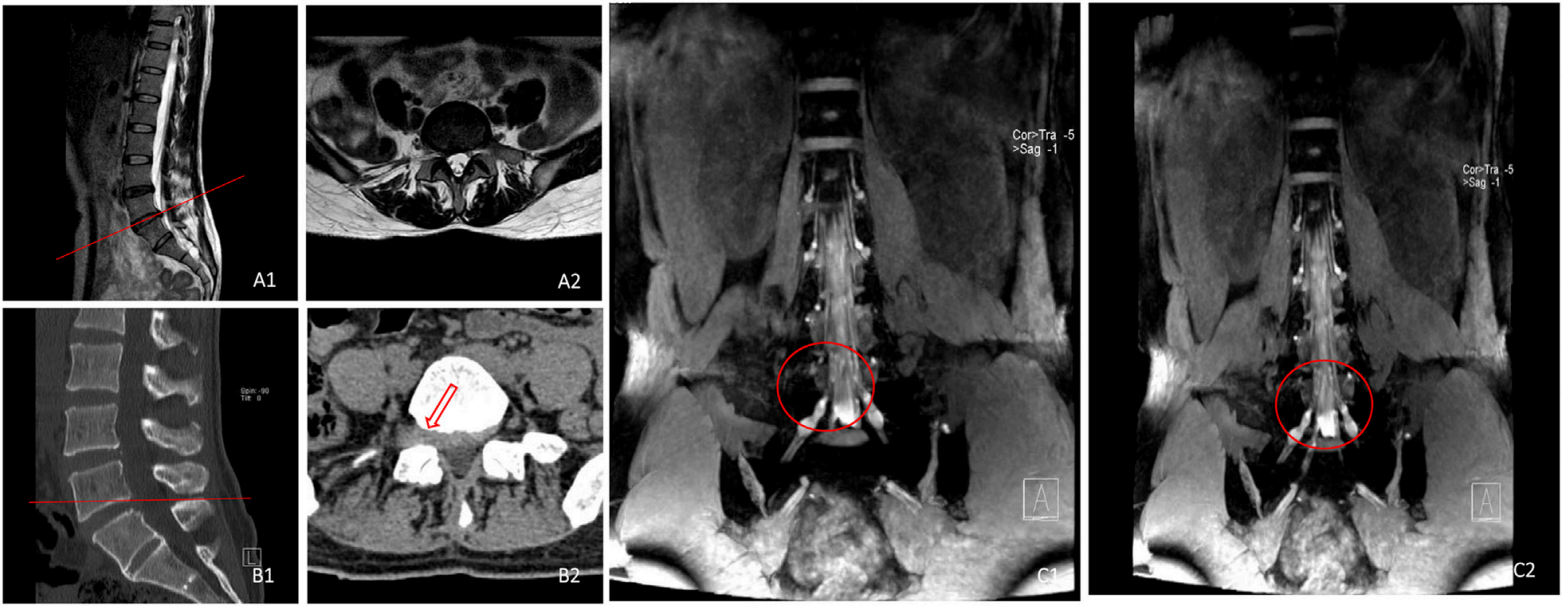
Sagittal (A1) and cross-sectional (A2) MR images indicated that the right side of L4/L5 intervertebral disc protruded, Sagittal (B1) and cross-sectional (B2) CT images displayed that the extreme lateral side of L4/L5 segment protruded greatly, and 3D-DESS image (C1, C2) revealed that the right L5 nerve root was compressed in the spinal canal, and the walking was interrupted. The protruded intervertebral discs on the extreme lateral side did not compress L4 nerve root, with smooth walking.
